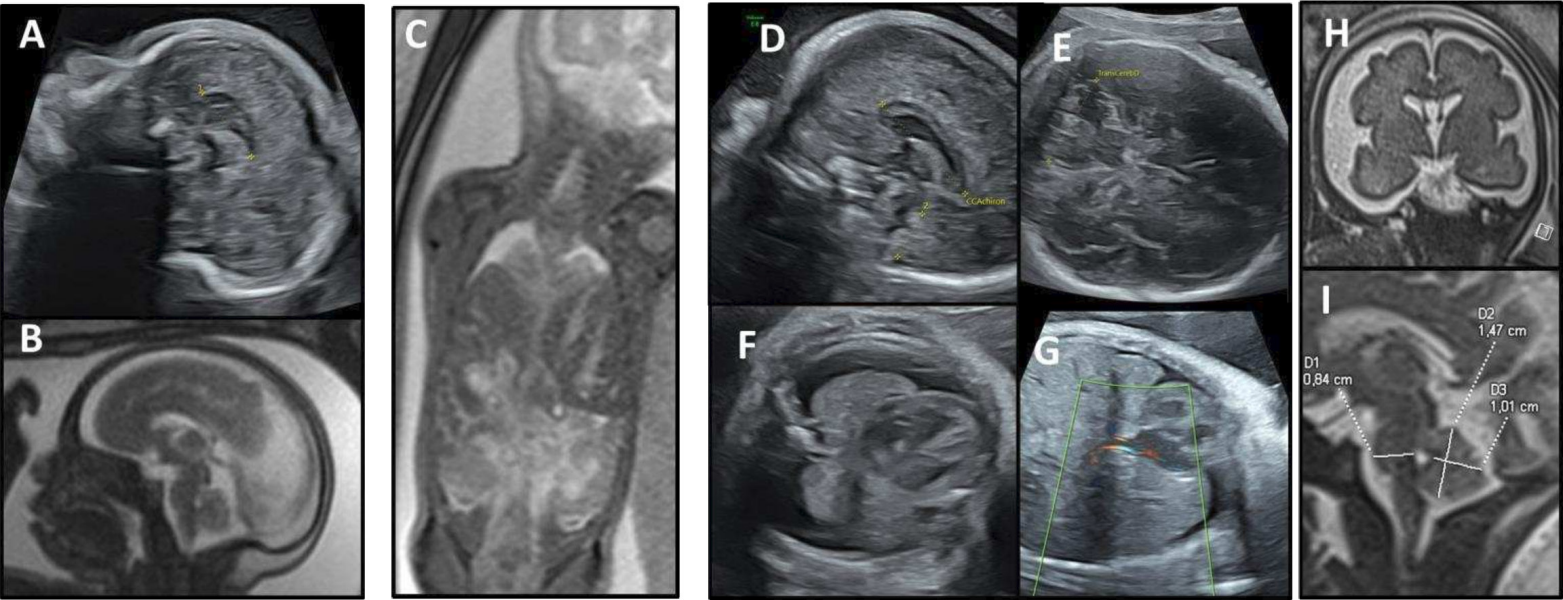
Prenatal imaging. Patient 5 (A) ultrasound at 24 GW then (B) MRI at 28 GW, both showing a short corpus callosum at the third percentile and the persistence of a nuchal thickening. Patient 6 (C) MRI at 29 GW, revealing a bilateral CDH, with intrathoracic right and left sides of the liver and an intrathoracic stomach. Patient 11 (D–I) showing at 27 GW ultrasound scans (D–G), a short corpus callosum at the third percentile (D), a cerebellar hypotrophy <1st percentile (E), a bilateral thoracic effusion (F) and a CDH with herniation of the right lobe of the liver (G). Fetal brain MRI at 30 GW (H,I) showing on coronal T2-weighted image a markedly enlarged subarachnoid space related to microcephaly (H), and on sagittal T2, biometries of the brainstem and cerebellum bellow the third percentile, supporting pontocerebellar hypoplasia (I). CDH, congenital diaphragmatic hernia; GW, gestational weeks.
